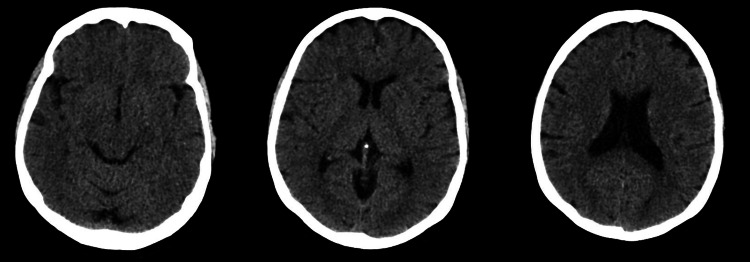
CT head. Multi-slice unenhanced CT images of the head. Normal study, with no evidence of acute intracerebral haemorrhage, major territorial infarct, space-occupying lesion, or hydrocephalus. CT: computed tomography.
